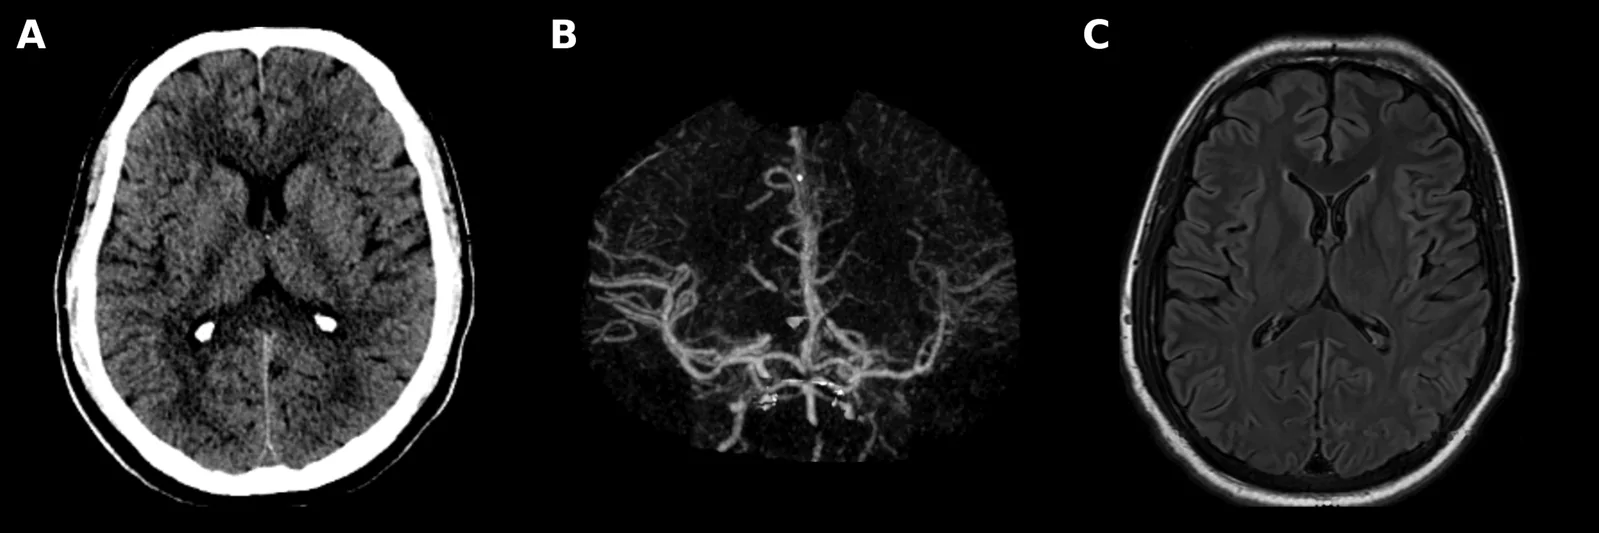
Representative admission neuroimaging (A) Axial non-contrast CT brain showing no acute intracranial abnormality. (B) CT angiography maximum-intensity projection showing no cerebral large-vessel occlusion. (C) Axial fluid-attenuated inversion recovery MRI showing no focal acute parenchymal abnormality. CT, computed tomography; MRI, magnetic resonance imaging.
